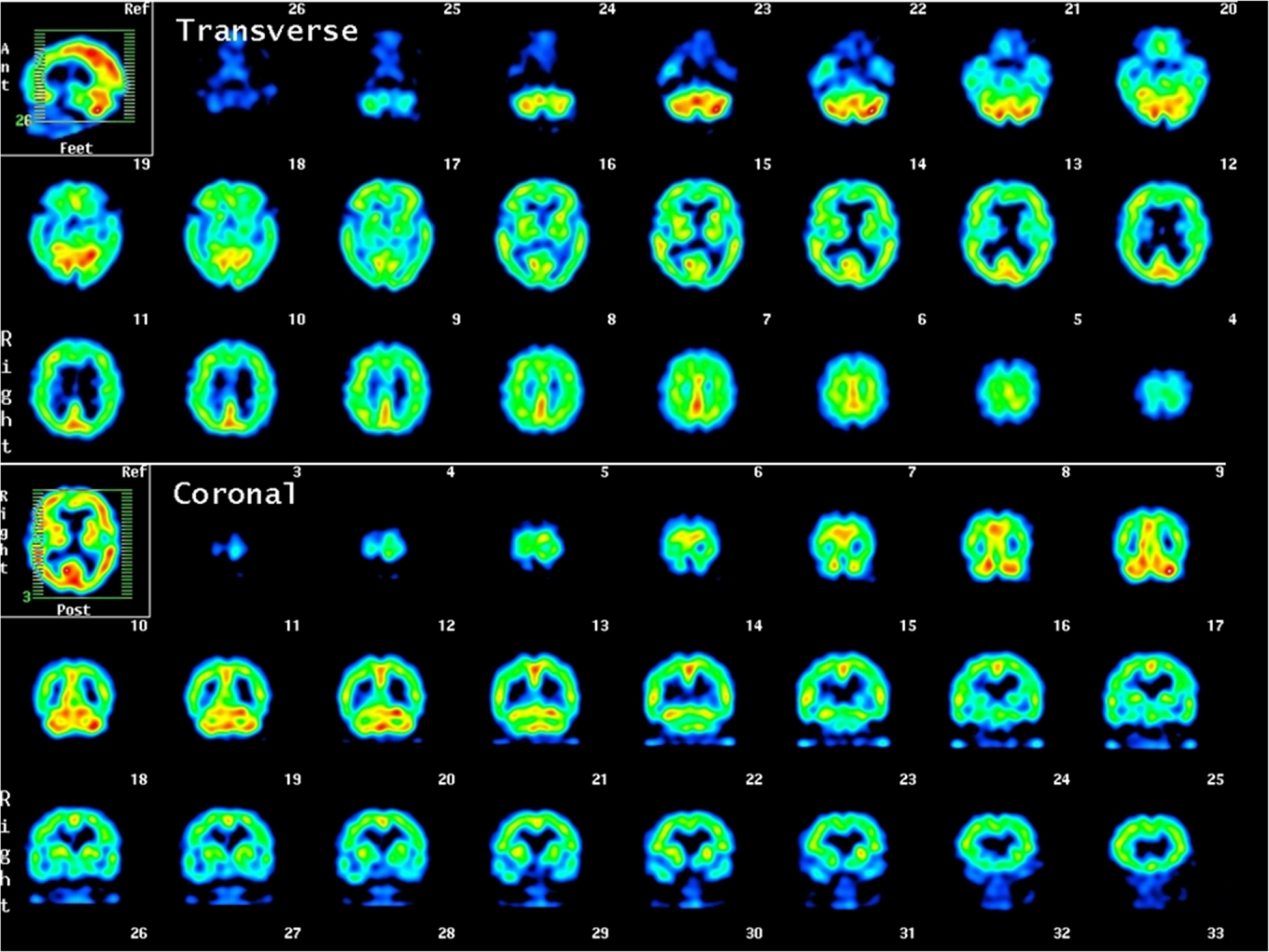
Single-photon emission computed tomography revealed areas of relatively decreased blood perfusion around the Sylvian fissure, while increased blood perfusion in the external layer around the convexity side
